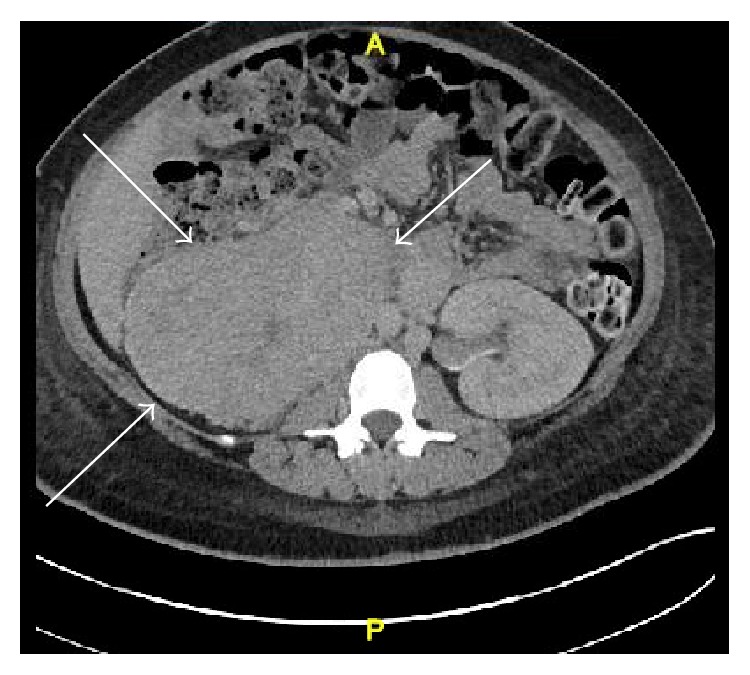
CT scan abdomen/pelvis with contrast showing large retroperitoneal mass.
